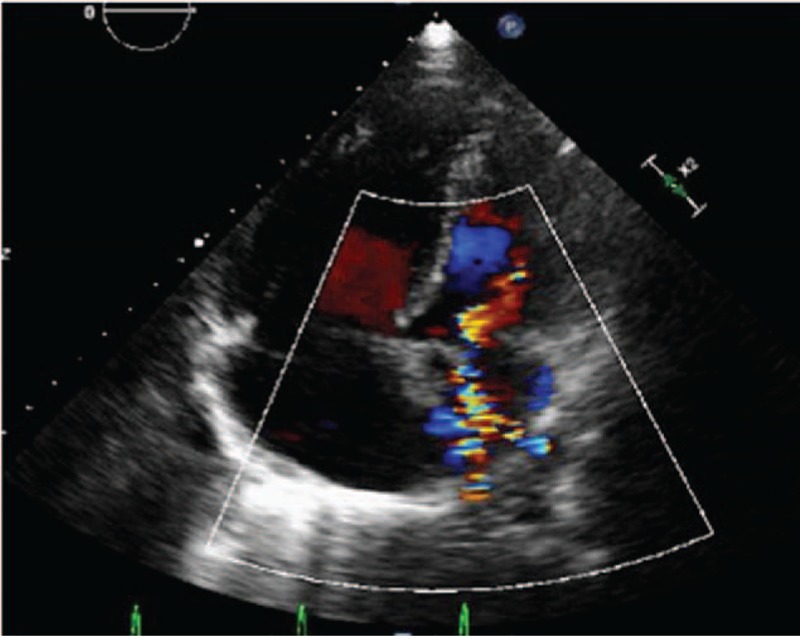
Four-chamber echocardiography view of PV stenosis in patient with FM. Note the presence of turbulent flow within the left atrium.
